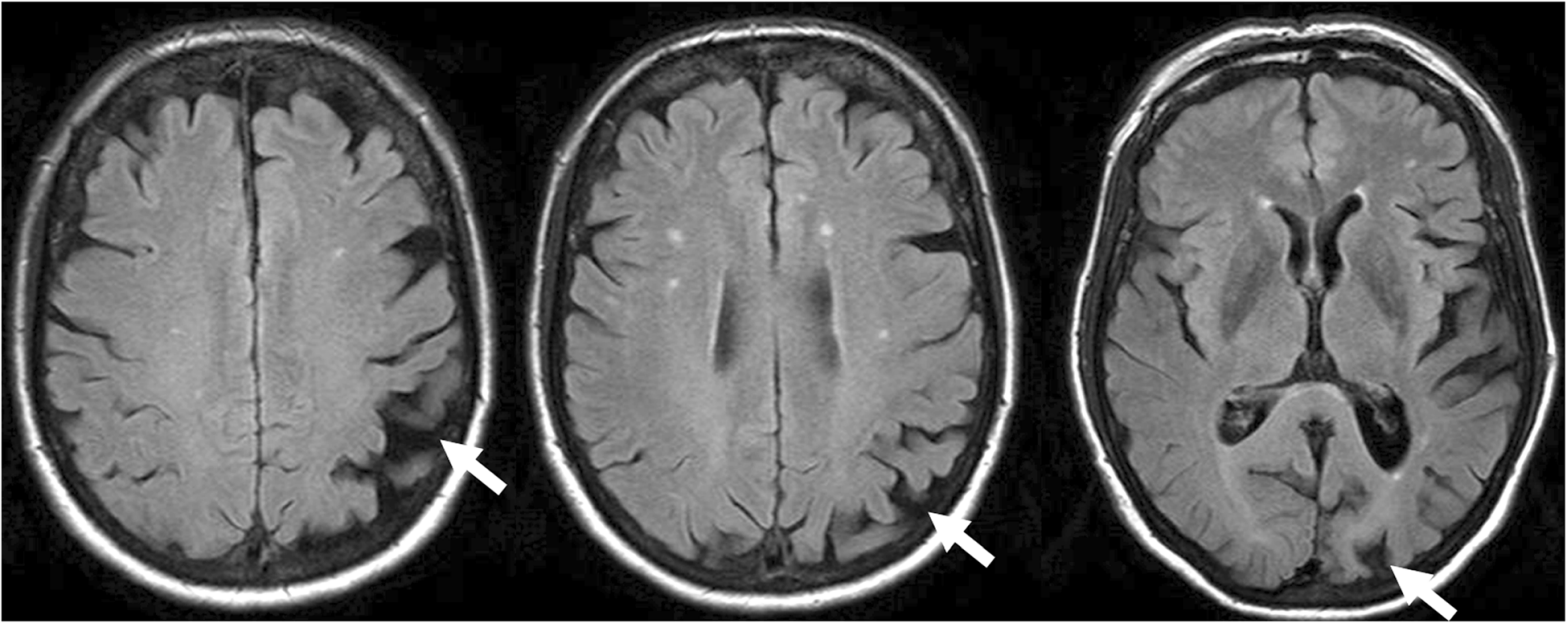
Patient 9. Cerebral MRI (T1) showed a left occipito-parietal atrophy (arrows)
